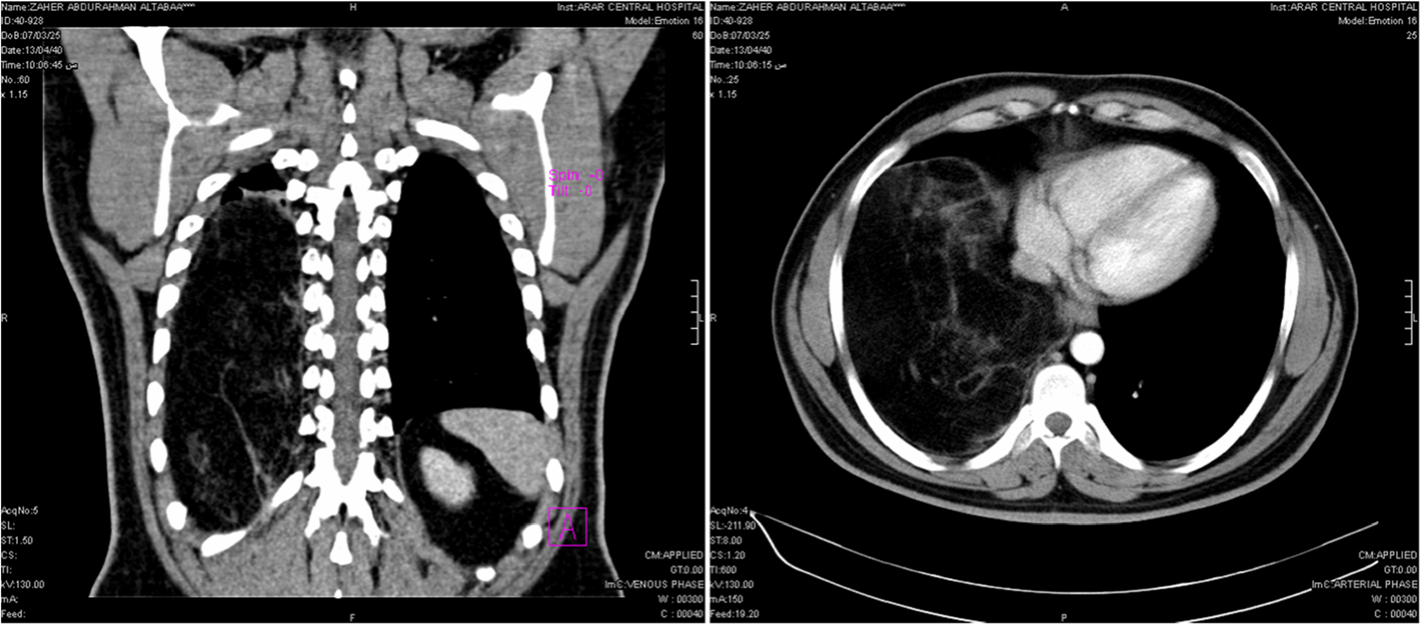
CT scan chest with contrast showing a well-defined huge intrathoracic mass
